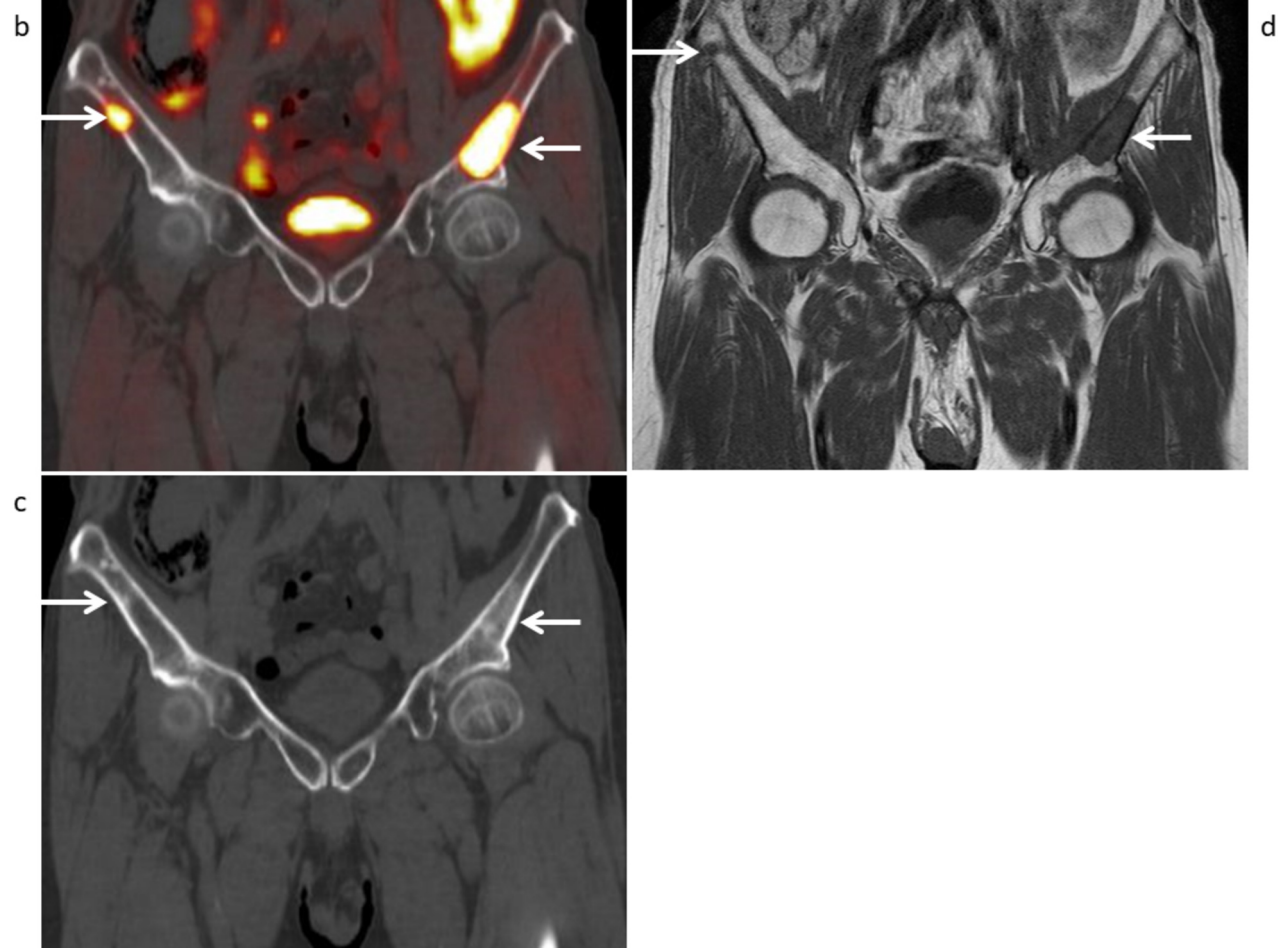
Coronal 11C-choline PET/CT and pelvic MRI (Case 2) (b) Coronal ^11^C-choline PET/CT and (c) CT part (bone condition) show strong ^11^C-choline uptake (SUVmax 11.32 (left) and 5.91 (right)) of the osteoblastic change in the bilateral iliac bones (arrows), with a score of 5 for diagnosing bone metastasis. (d) Coronal T1-weighted MR image shows hypointense areas in the bilateral iliac bones (arrows), with a score of 5 for diagnosing bone metastasis.
